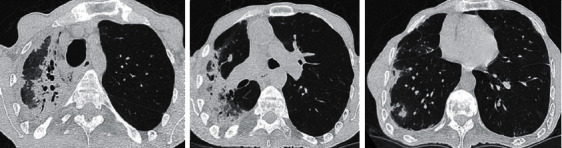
Thorax HRCT performed in 2019. It shows chronic consolidation with traction bronchiectasis compatible with fibrosis in the apical and posterior segments of the upper lobe which is extending to the superior segment of the lower lobe. Nodular alveolar densities in the lateral basal segment of the right lower lobe are also seen.
